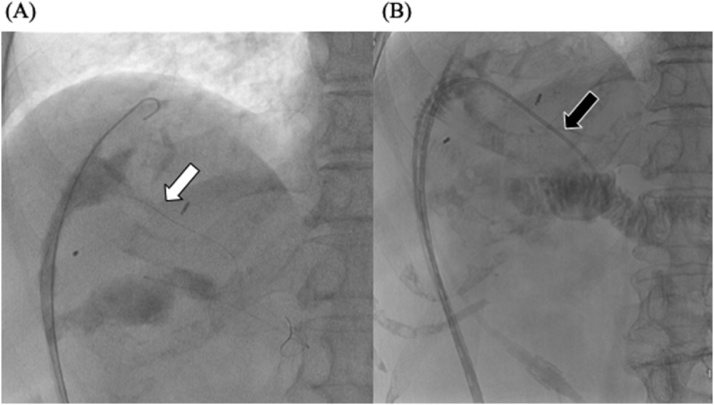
X-ray fluoroscopic examination on the 28^th^ postoperative day, showing (A) the flow to the anterior branch of the right intrahepatic bile duct and the cannulated guide wire (white arrow). (B) The guide wire was advanced toward the jejunum via the junction of choledochojejunostomy under the assistance of 5Fr. Cobra-type catheter (black arrow).
